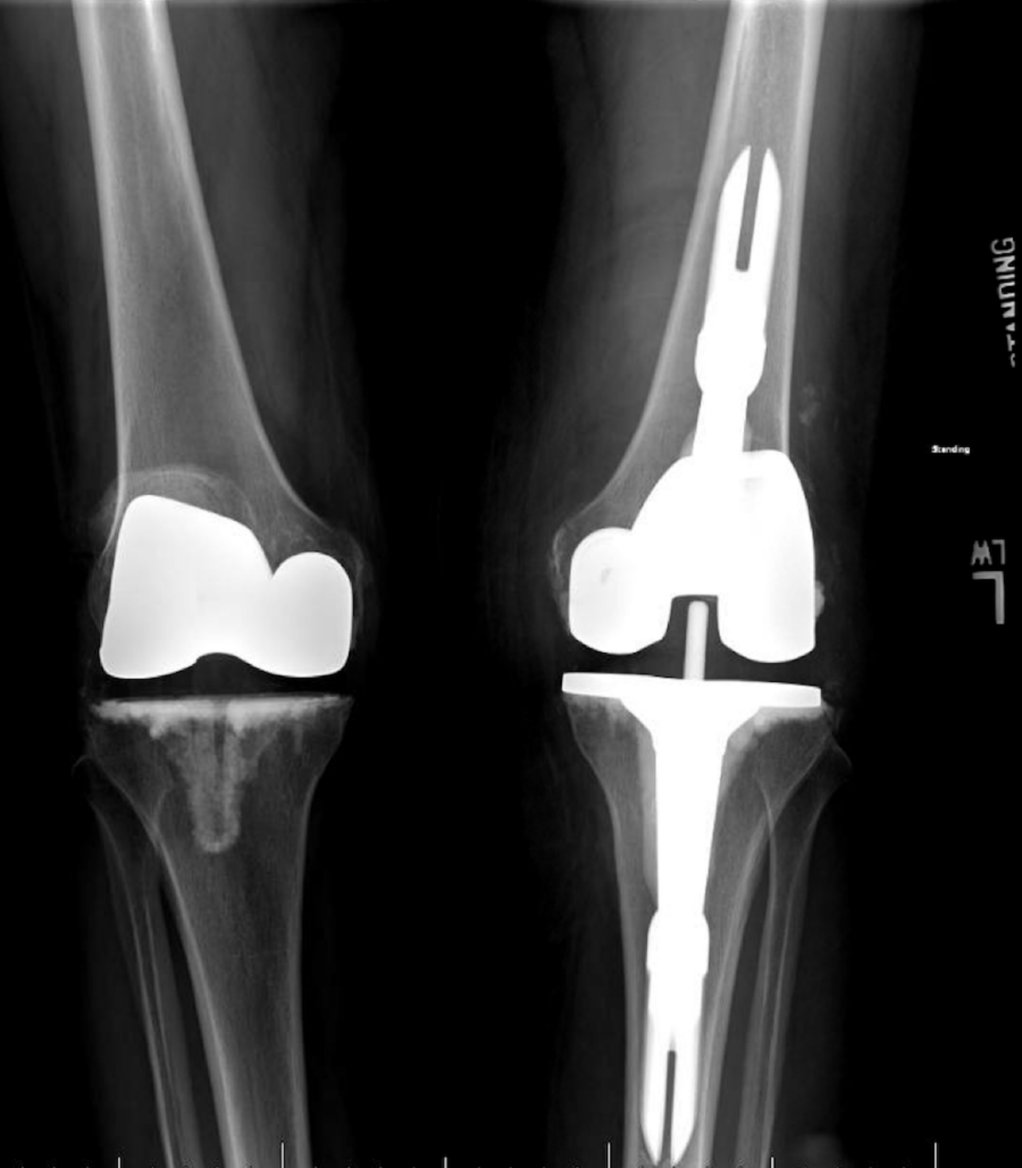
Postoperative radiograph at two-week follow up
